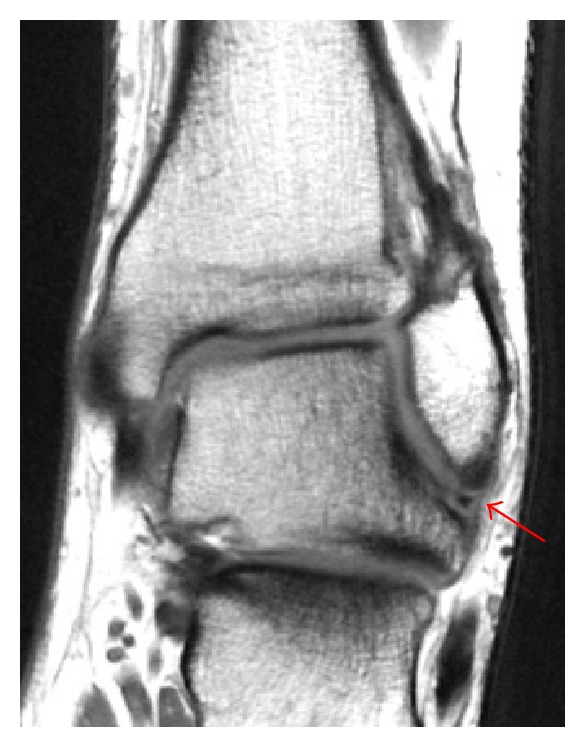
Coronal T1-weighted MR image showing an ATFL tear (arrow).
